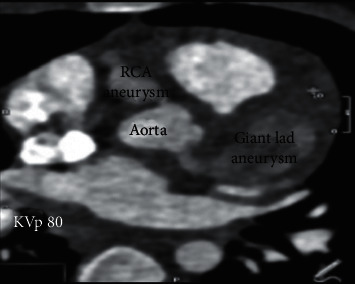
CT angiography showing massive LAD aneurysm.
